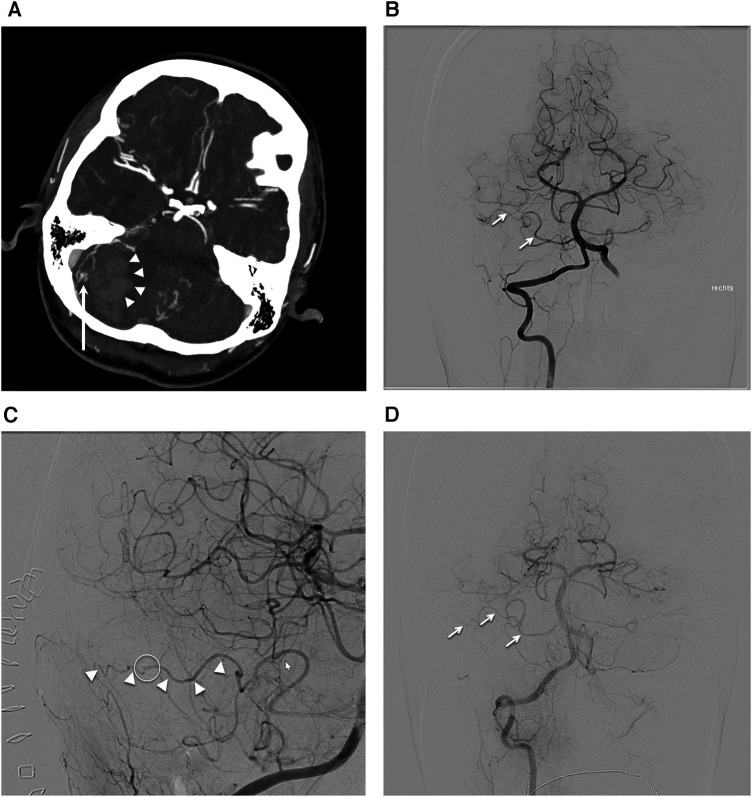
Case presentation. (A) Computed tomography angiography axial cut showing cerebral hematoma on the right-hand side (arrowheads) and associated arteriovenous malformation (AVM) nidus (arrow). (B) Anterior posterior projection of the preoperative digital subtraction angiography (DSA) after right vertebral artery injection displaying the AVM nidus being fed by the anterior inferior cerebellar artery (AICA) branches (arrows). (C) Oblique projection of the postoperative DSA after right vertebral artery injection showing the intact *en-passant* AICA (arrowheads) and the AVM clip on the previous AVM feeder (circle). (D) Anterior posterior projection of the DSA after right vertebral artery projection highlighting the intact *en-passant* AICA (arrows).
